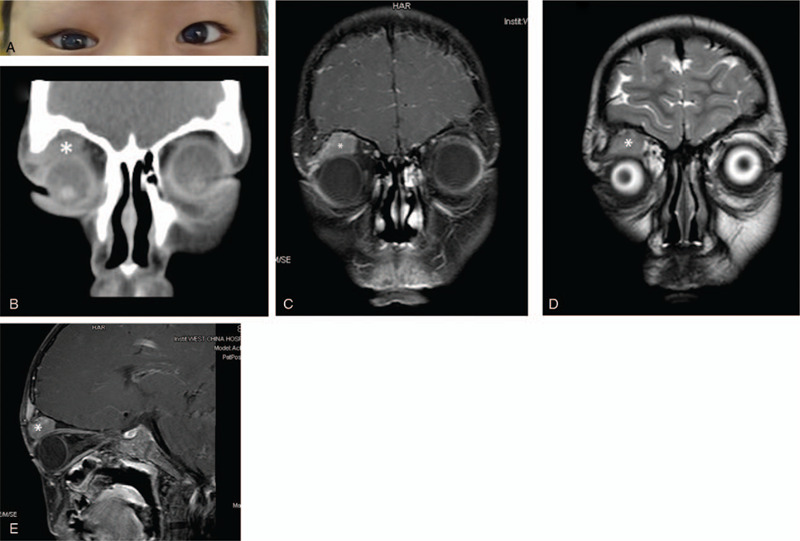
A. The appearance of the eyes with right orbital tumors. B. CT scans revealing the location of the orbital tumor (white asterisk) in the superior orbit. C and D. Coronal T1-weighted MR image of the orbital tumor (white asterisk) (C) and Coronal T2-weighted MR image of the orbital tumor (white asterisk) (D). E. Sagittal T1-weighted MR image of the orbital tumor (white asterisk).
